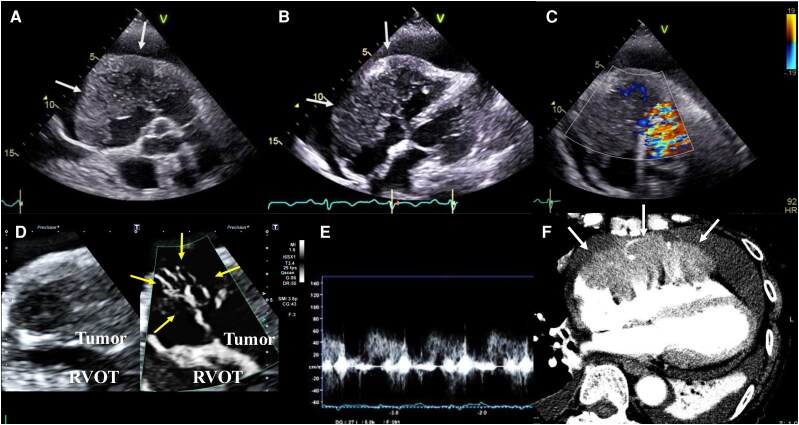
A presumed case of cardiac sarcoma. Transthoracic echocardiography scans in (*A*) a short-axis view and (*B*) a modified apical four-chamber view of the right ventricle showing intracardiac tumours with irregular margins in the right ventricular outflow tract, the free wall of the right ventricle, and the epicardium (white arrows). (*C*) Conventional colour Doppler with reduced velocity scale could not adequately display the intratumoural blood flow signal. (*D*) Superb microvascular imaging detected abundant intratumoural blood flow signals (yellow arrows). (*E*) Flow pattern in the tumour depicted using pulse Doppler: the blood flow signals are predominantly seen in diastole, similar to the pattern seen in a coronary artery. (*F*) Contrast-enhanced cardiac computed tomography scan showing contrast effects in the tumour (white arrows).
